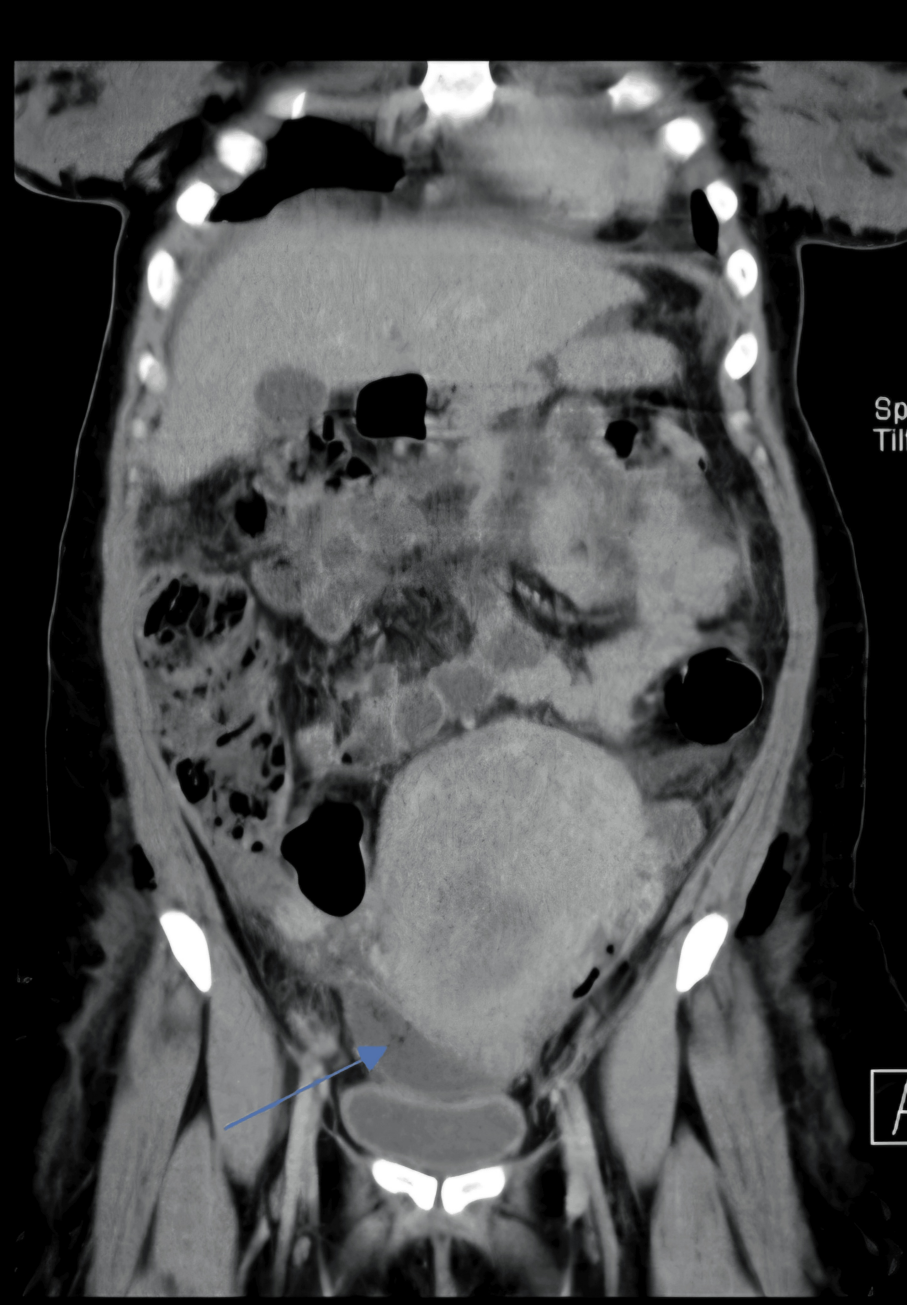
Computed tomography (CT) pelvis and abdomen showing hematoma at surgical site
